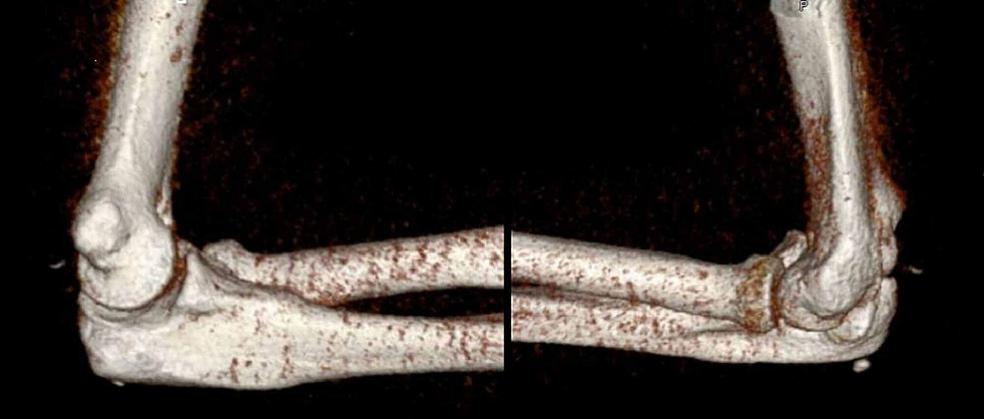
3D reconstruction of the elbow from the CT scan. 3D, three-dimensional
